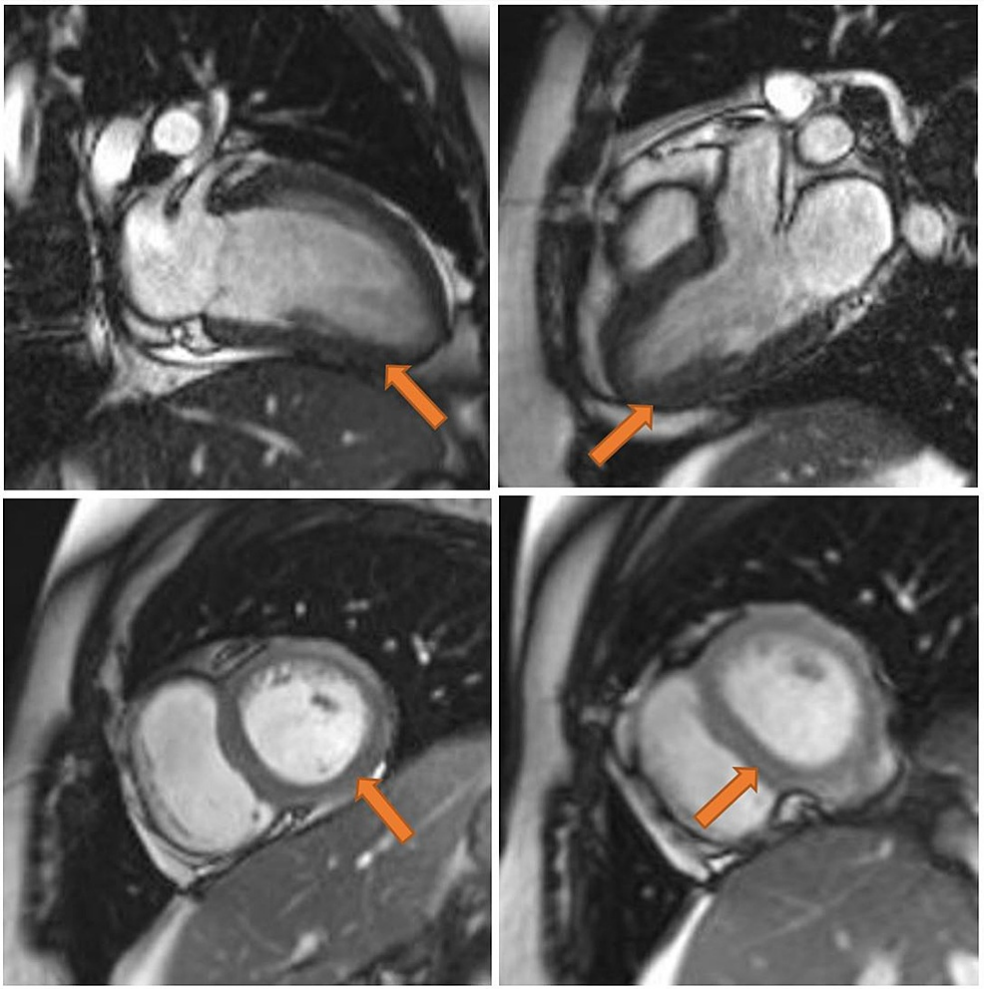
Cardiac MRI There is diffuse left ventricular myocardial edema with associated subpericardial enhancement pattern on delay acquisition. There is also severe global mild hypokinesia with no evidence of pericarditis or pericardial effusion.
